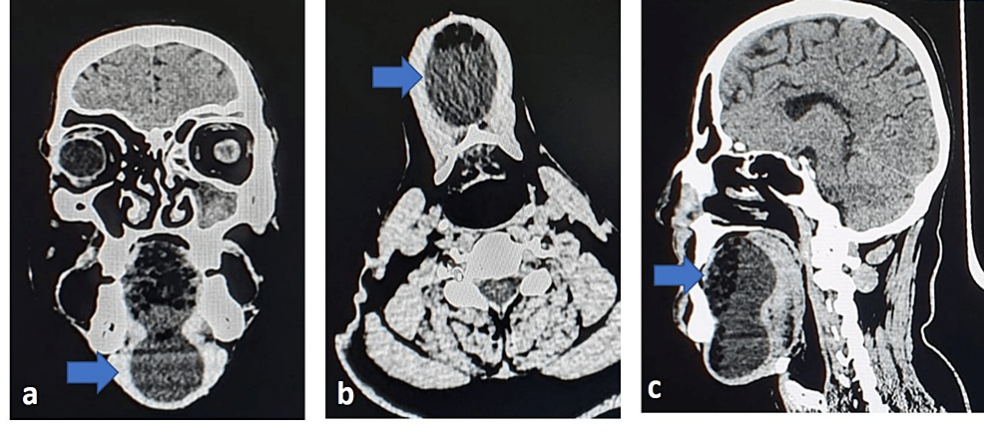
Computed tomography images of the lesion a) Coronal view showing dumbbell shaped lesion with multiple fat densities b) Axial view showing the inferior bulge with multiple fat densities c) Sagittal view showing dumbbell shaped hypodensity with multiple fat densities in the anterior portion
